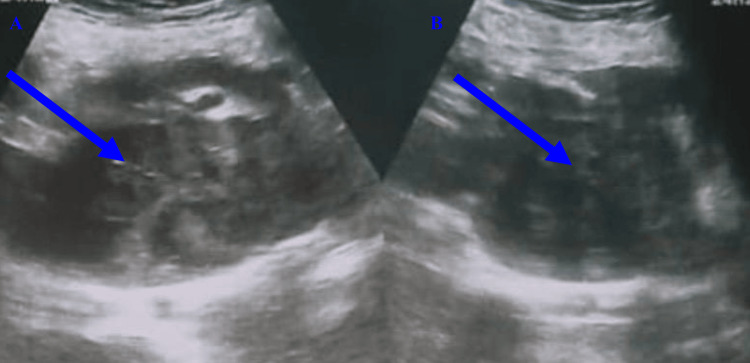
Ultrasound image of the patient (A) Mass showing internal echogenic foci suggesting calcification. (B) Cross-fused kidney in the left iliac fossa showing a heterogeneous, predominantly hypoechoic mass
